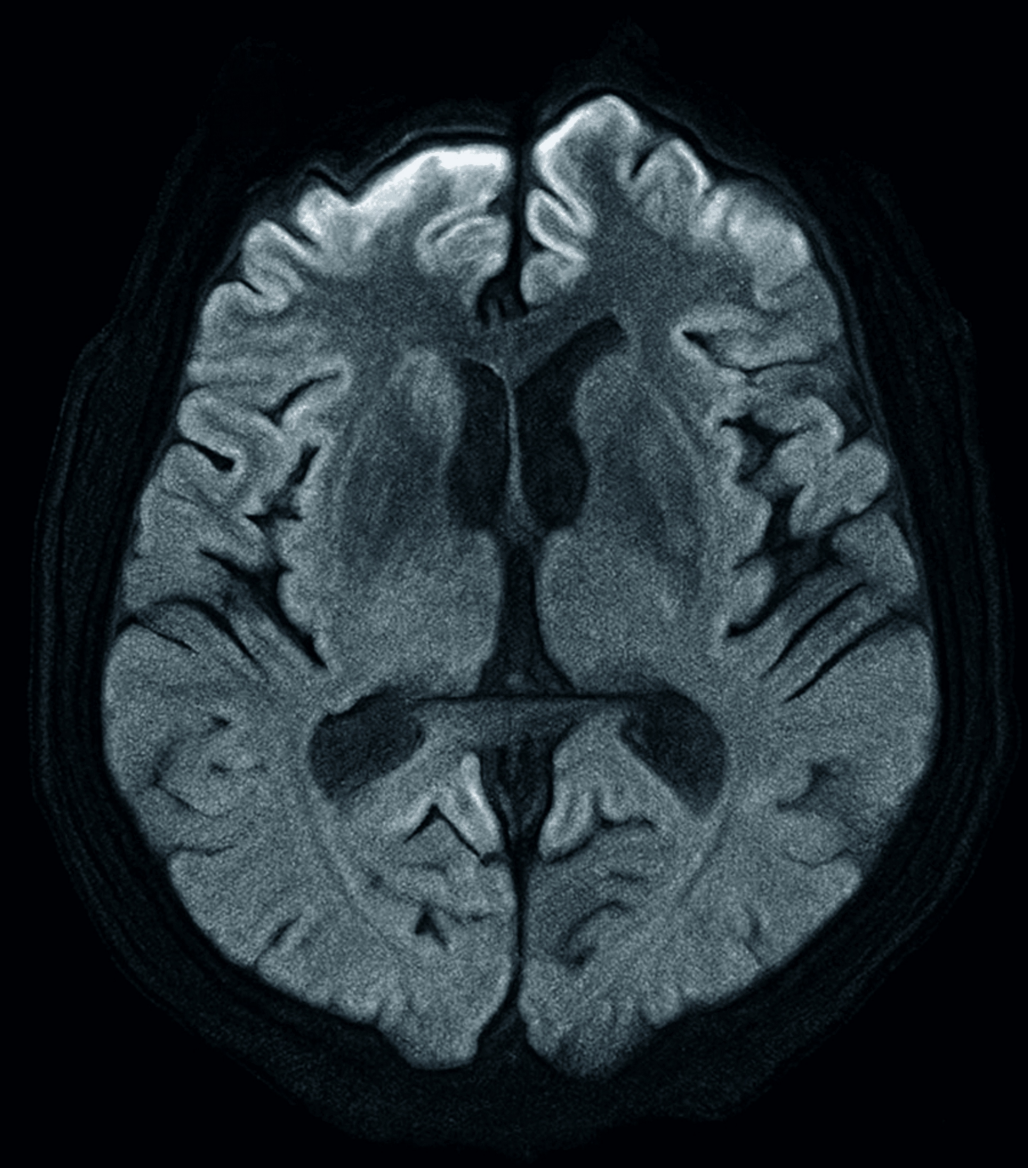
MRI brain ​​​​​​​diffusion-weighted imaging (DWI) and FLAIR sequence shows diffusion restriction in the head of the caudate nucleus, along with the characteristic cortical “ribboning appearance.” FLAIR: fluid-attenuated inversion recovery
